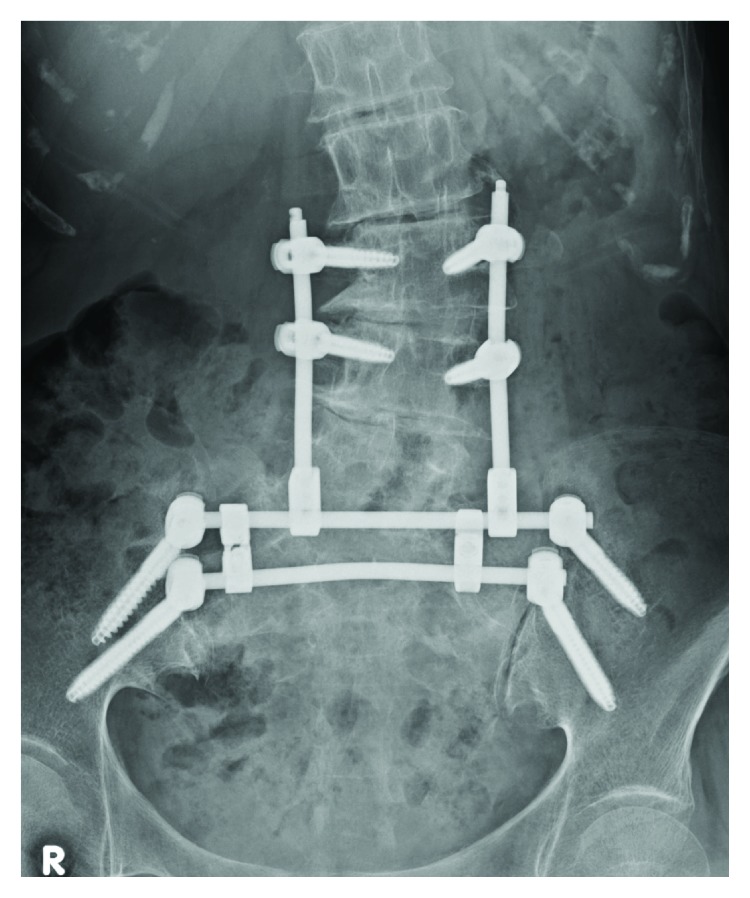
Postoperative anteroposterior radiograph. Percutaneous pedicle screws were inserted in L3 and L4, and 2 iliac screws were inserted on each side. Bilateral iliac screws were connected by 2 transverse rods and a crosslink. The crossing points of rods for lumbar pedicle screws and the cranial transverse rod for iliac screws were connected by rod connectors, completing lumbopelvic fixation.
